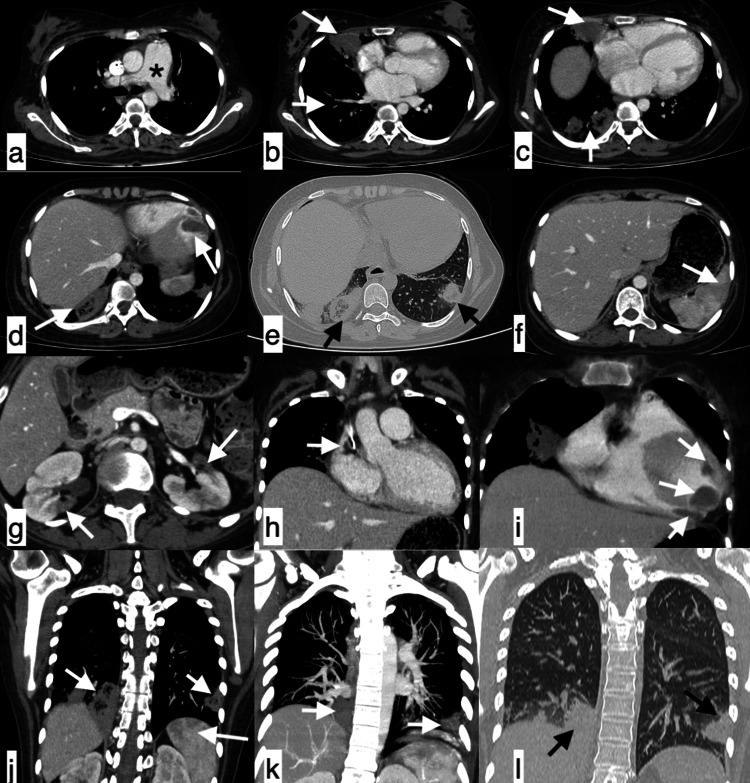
Antiphospholipid syndrome An 18-year-old female patient with antiphospholipid syndrome secondary to SLE presented with shortness of breath and chest pain of two-day duration. CT pulmonary angiogram revealed a dilated main pulmonary artery (a, asterisk) with thrombosis of subsegmental vessels (b, white arrow), with resultant infarcts seen as areas of wedge-shaped consolidations with a broad base toward the pleura, along with a few air bronchograms within the medial segment of the right middle lobe. Areas of ground-glass opacities with surrounding consolidation extending to the subpleural location showed a reverse halo sign in the bilateral lower lobes (b-e, j-l, white and black arrows). Additional findings included a large eccentric filling defect in the distal SVC around the central line (h, white arrow), a non-enhancing thrombus in the left ventricle and right ventricle near the apex (i, white arrows), cardiomegaly with left ventricle and left atrium dilation (c), splenic infarcts (f, j, white arrows), and renal infarcts (g, white arrows). SLE: systemic lupus erythematosus; SVC: superior vena cava
